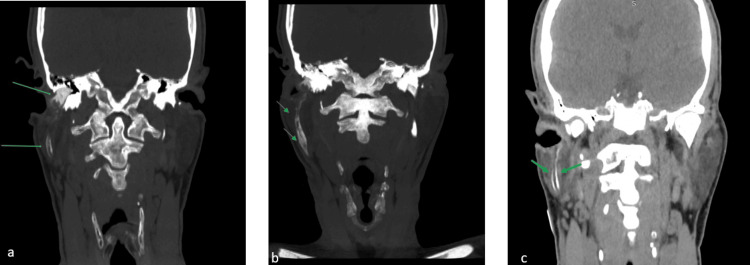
CT fistulography The green arrows depict a fistula with a maximum diameter of 1.6 cm, starting from the external auditory canal, continuing through the parotid tissue, and ending in the right submandibular skin region (a, b, and c show the coronal view).
